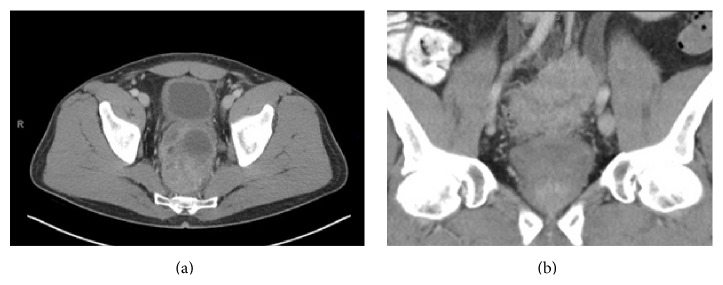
CT imaging of pelvis: (a) axial images showing large mass posterior to the bladder with areas of necrosis; (b) sagittal images with mass superior to bladder extending to involve the left ureter with proximal hydroureteronephrosis.
